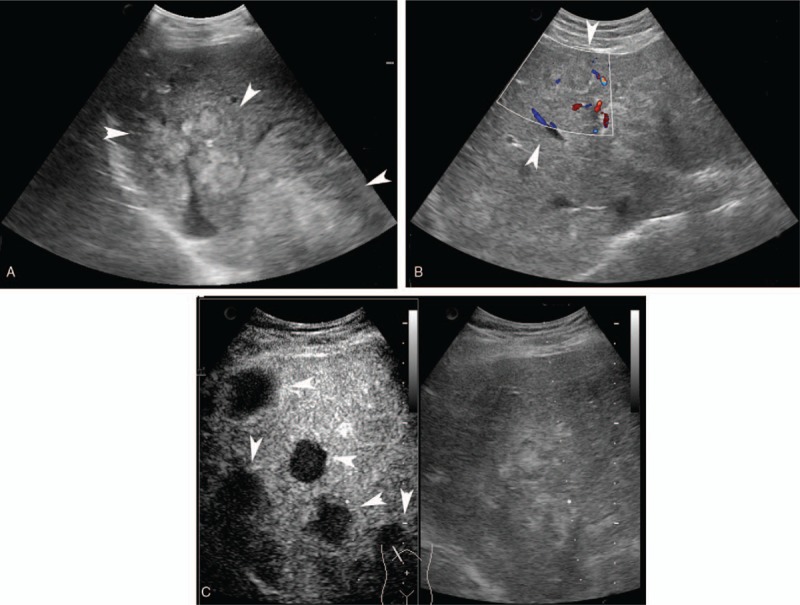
A 48-year-old man with hepatic AE. A: Multiple hyperechoic irregular lesions (arrow head) were found in the right hepatic lobe on US; B: No signal in the lesion (arrow head) was detected using color Doppler mode in the left hepatic lobe. C: CEUS showed multiple lesions (arrow head) with no enhancement in the nodules in the arterial phase which was significantly different from that seen on US. The characteristics of the sonographic image were similar to a “black hole”. AE = alveolar echinococcosis, CEUS = contrast enhanced ultrasound, US = ultrasound.
